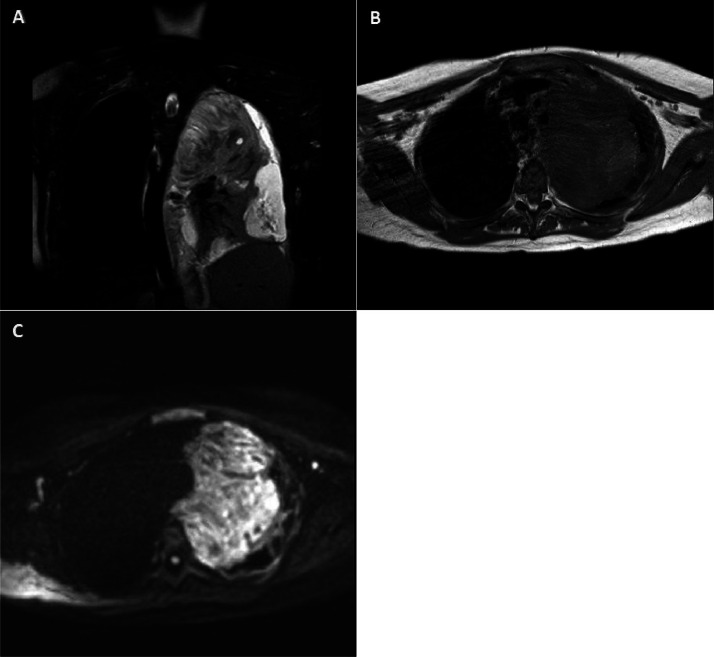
(A) Coronal T2W-SPAIR, (B) axial T1W, and (C). axial DWI sequences show large lobulated Mass with high T2W and iso to low T1W signal intensity measuring 140 × 100 × 95 mm with diffusion restriction in the Left side of the mediastinum with extension to the left hilum and upper and lower lobe.
